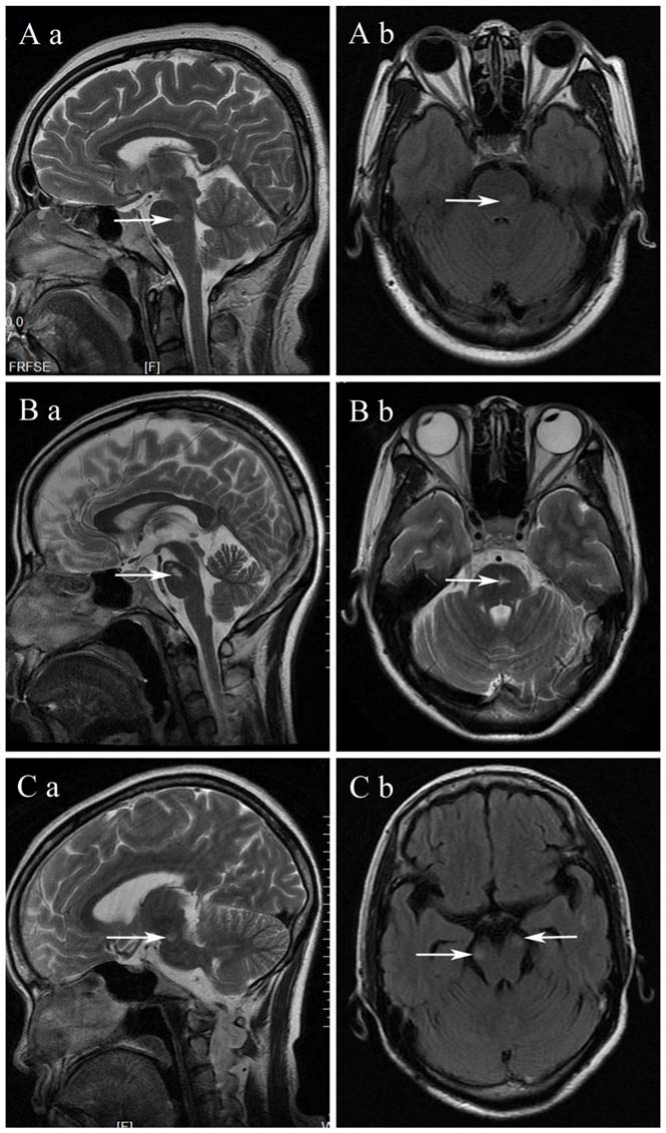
Brain stem lesions (arrows) on MRI of multiple sclerosis (MS). (A, B) MRI showing solitary pons lesion with sharply defined borders. (C) MRI showing midbrain lesions.
